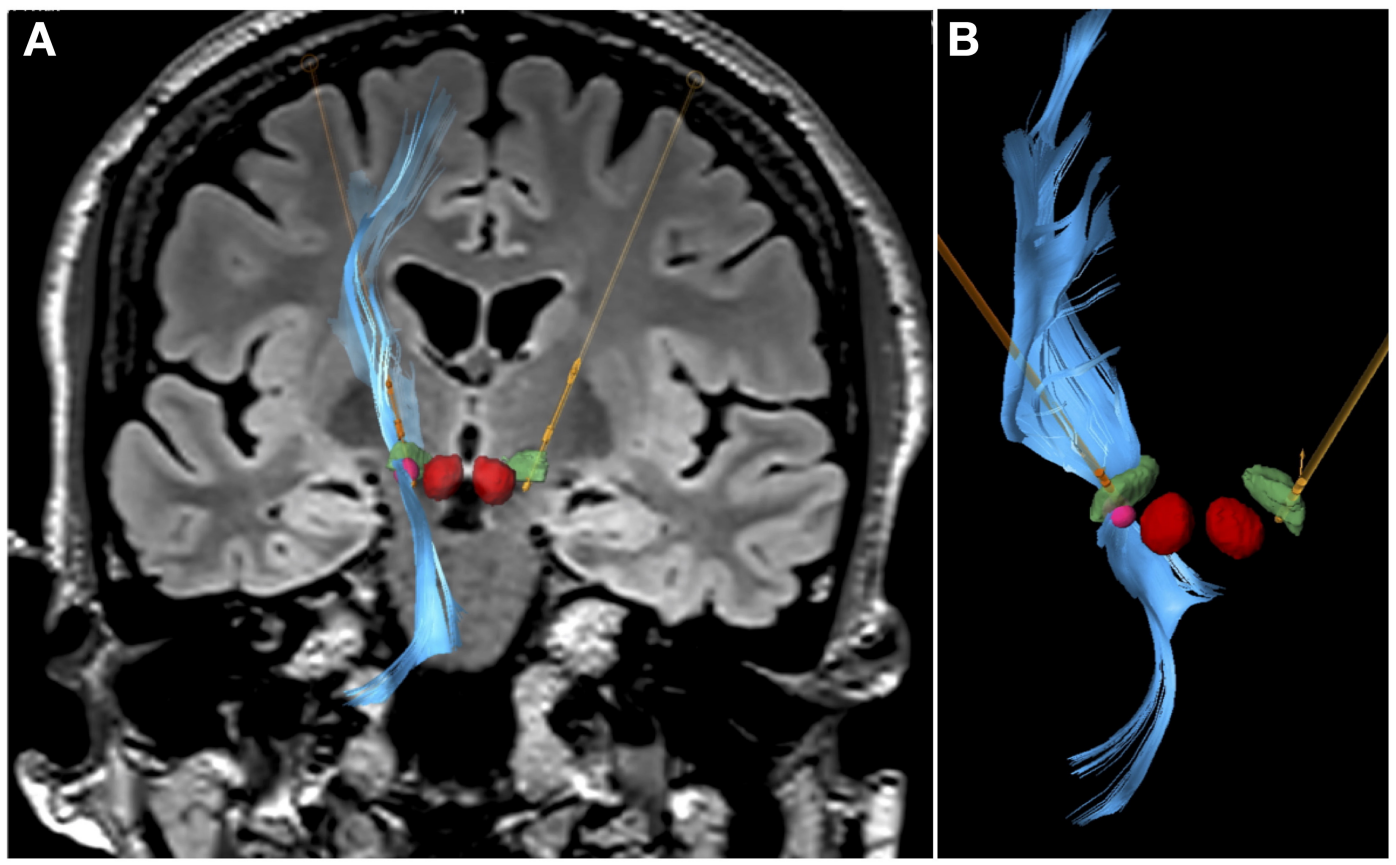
Tractography study. Representation of the associative fibers stimulated when contact 3 in the left STN was activated at 1.5 mA, 60 μs, and 130 Hz. (A) Coronal flair 3T MRI view, with segmentation of bilateral red nucleus (red), bilateral STN (green), directional leads (orange), and VTA (pink sphere) generated with contact 3 stimulation. Fibers toward the prefrontal cortex in the left side are represented in blue. (B) Close view of the left directional lead, left STN and the fibers passing through the VTA generated (in contact 3) toward the frontal region. Images obtained with Elements^©^ software (Brainlab^©^) and Guide XT^©^ software (Boston Scientific).
